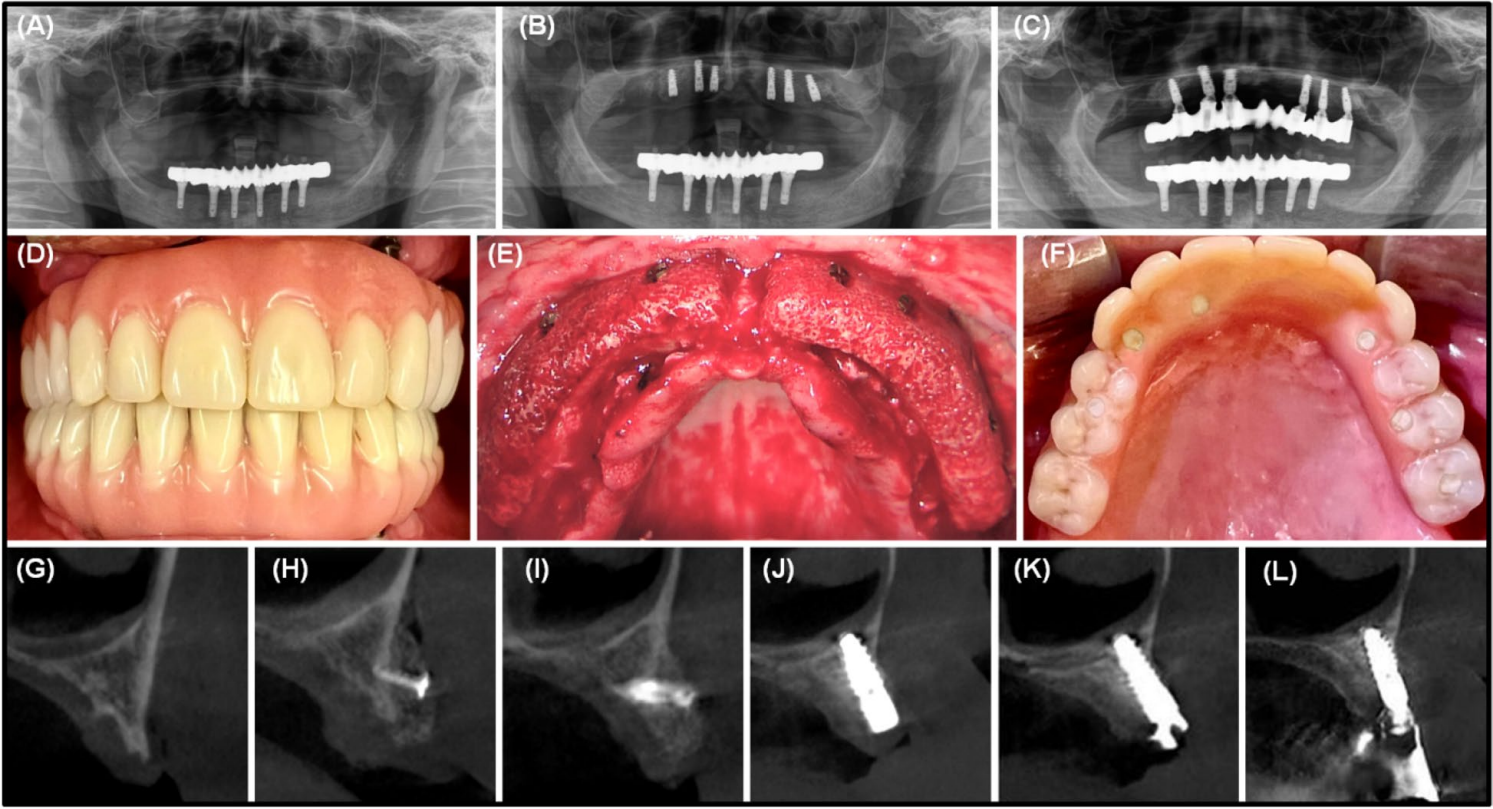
A OPT from the preoperative situation; B OPT after the implantation; C OPT shows the final screw-retained prosthetic construction; D clinical photo of the final rehabilitated maxilla (frontal view); E intraoperative image of two allogeneic individual 3D-bone blocks with simultaneous bilateral external sinus lifts for the augmentative rehabilitation of the upper jaw; F clinical photo of the finally rehabilitated upper jaw (occlusal view); G–L radiological chronology of augmentative, implantological, and prosthetic rehabilitation of a highly atrophic maxilla using combined therapy with external sinus lifts and 3D-milled bone blocks over a period of 19 months: G CBCT before augmentation (3 months); H CBCT after augmentation OP; I CBCT after augmentation (3 months); J CBCT after augmentation (12 months); K CBCT after augmentation (19 months); and L CBCT after augmentation (26 months)
